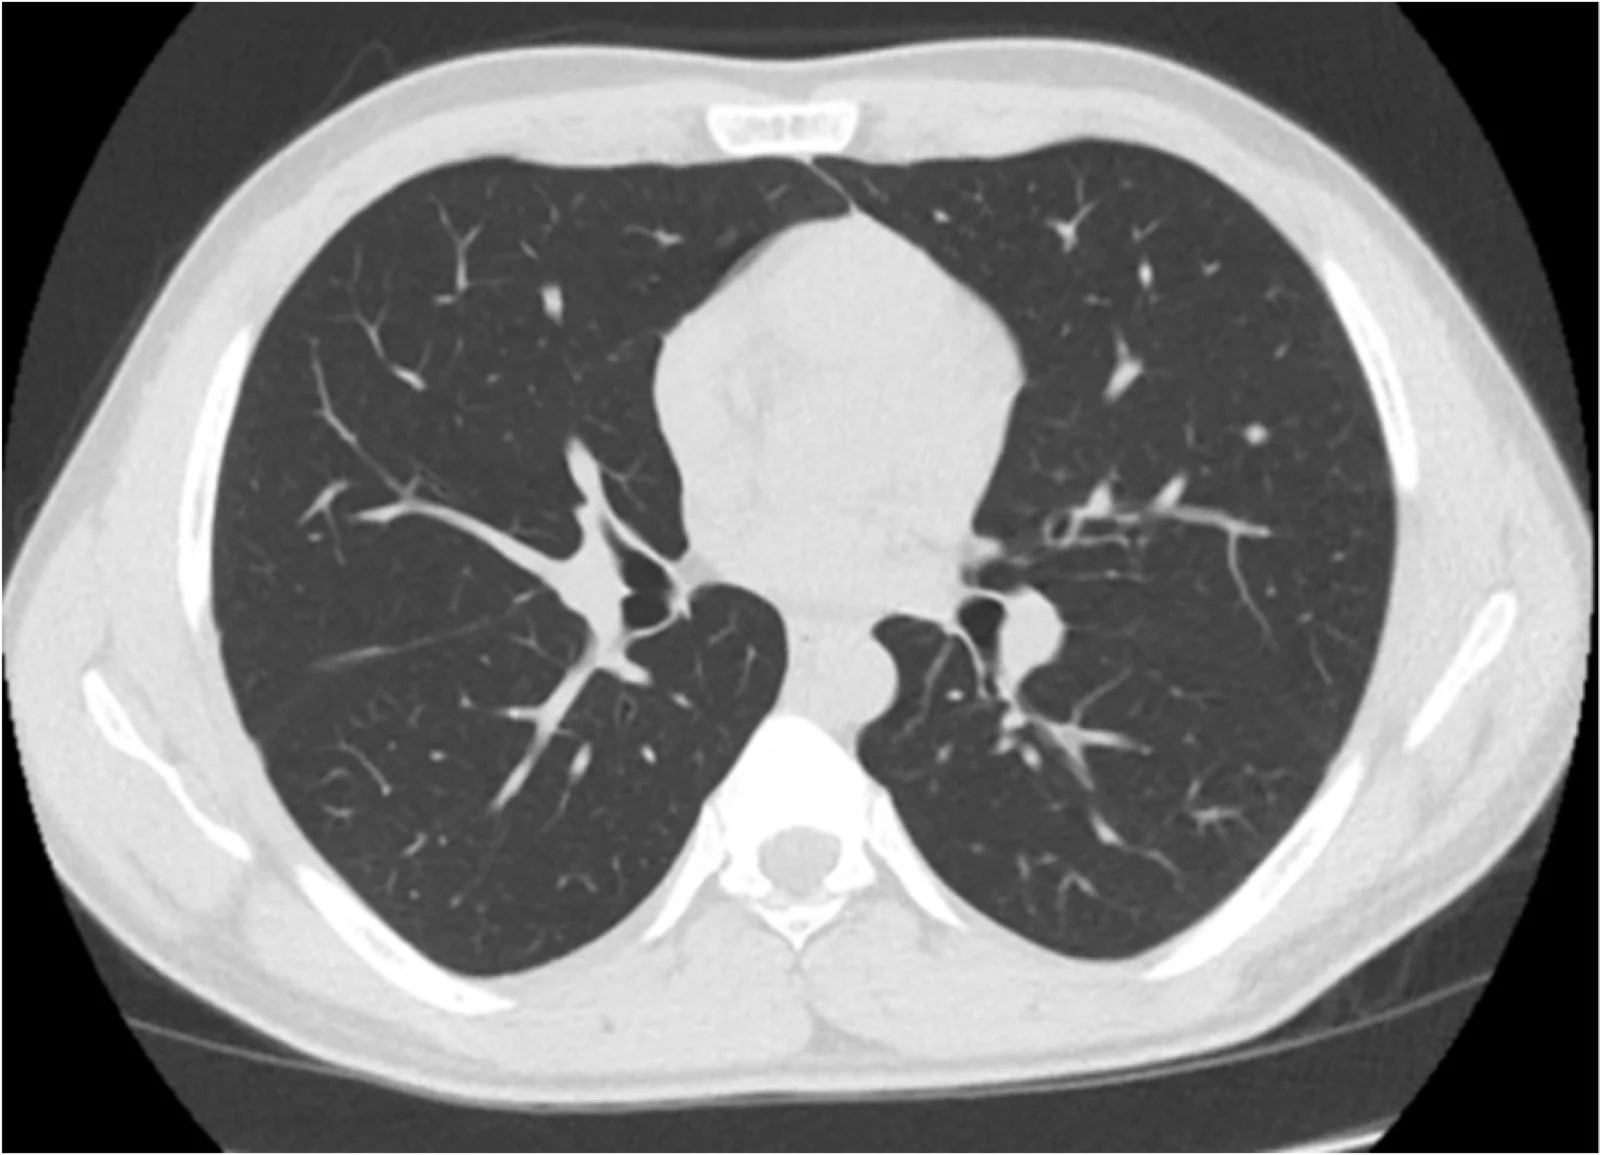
A 21-year-old male patient with a positive RT-PCR test, but no pulmonary infection is seen in the CT images (score 0). (Courtesy: Government Base Hospital, Almora, Uttarakhand).
